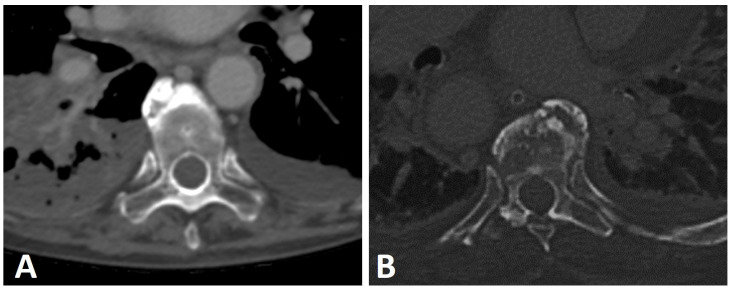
Axial CT images of two patients with thoracic DISH (not shown). (A) left-sided and (B) right-sided aortas with an anterior osteophyte located contralateral to the aorta’s position.
